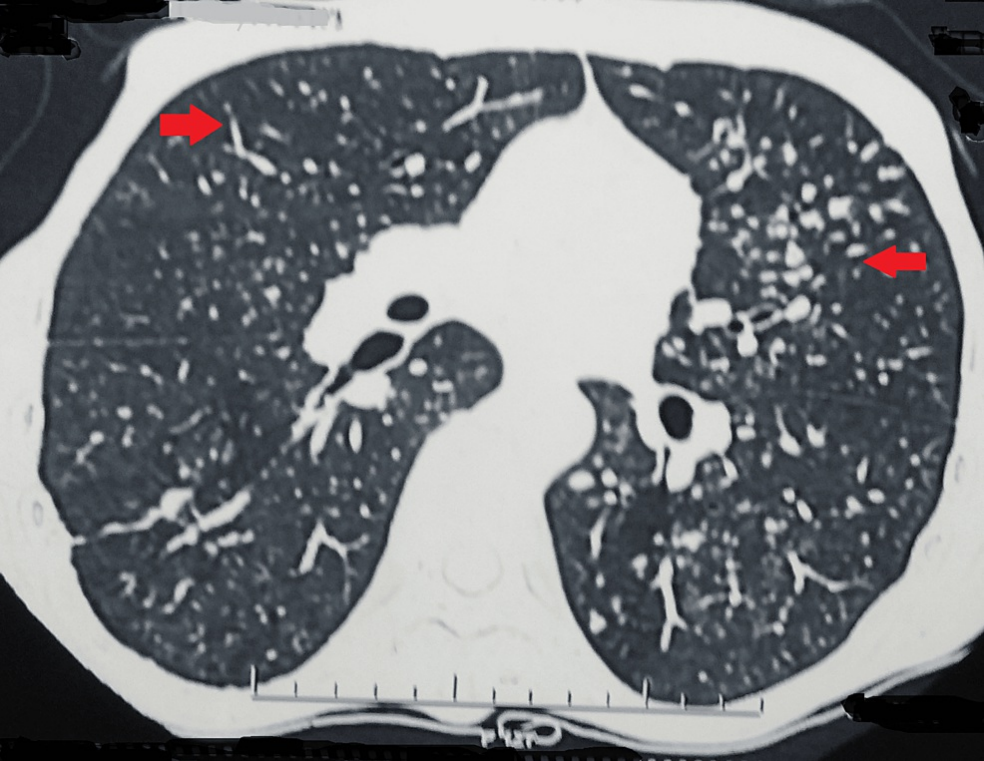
Computed tomography pulmonary angiogram showing bilateral involvement of the lungs
